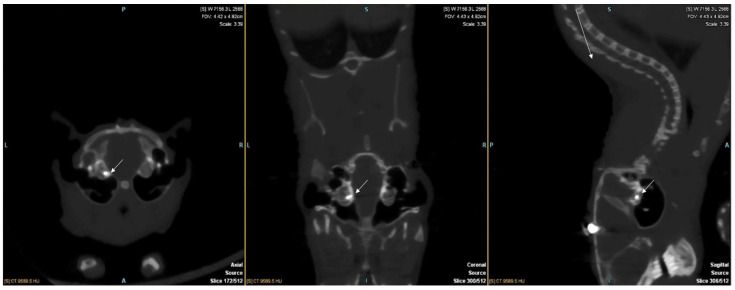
Axial, coronal, and sagittal slice reconstruction of a gerbil’s CT scans at 10 weeks after implantation. The 2 electrodes are visualised in the right cochlea (arrows).
